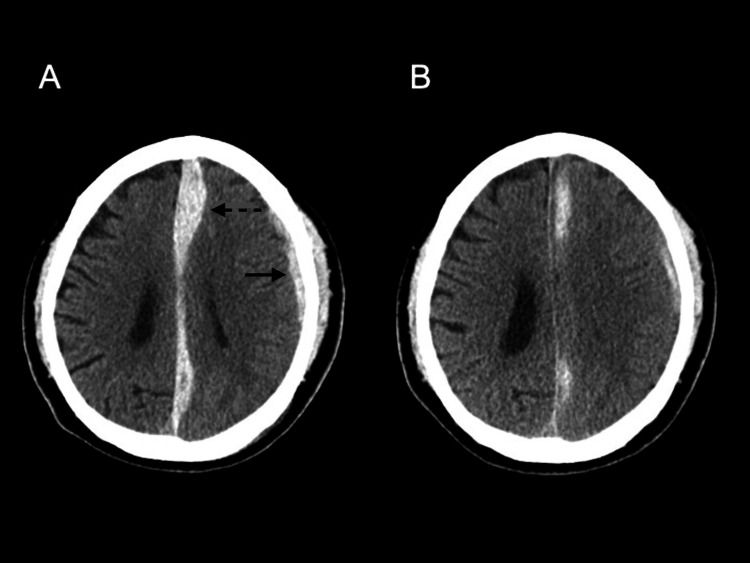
Initial head CT. (A) Acute subdural hematoma located along the falx cerebri (dotted arrow) and in the left frontotemporal region (solid arrow). (B) Increased cerebral edema with mass effect.
